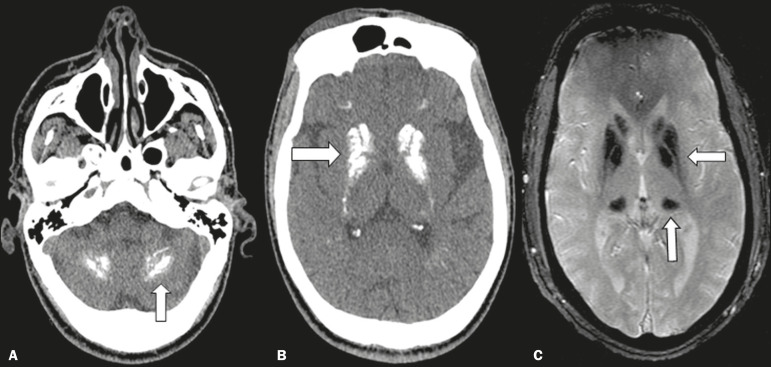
Unenhanced axial cranial CT scans, with soft-tissue window settings (A,B), and axial T2*-weighted gradient-recalled echo MRI sequence of the skull (C), showing confluent calcifications in the region of dentate nuclei (arrow) in a case of pseudo-hypoparathyroidism (A), confluent calcifications in the area of basal ganglia (arrow) in a case of Fahr’s disease (B), and areas of markedly hypointense signals, corresponding to calcifications in the region of the basal ganglia and pulvinar of the thalamus (arrows), in a case of Fabry disease (C).
